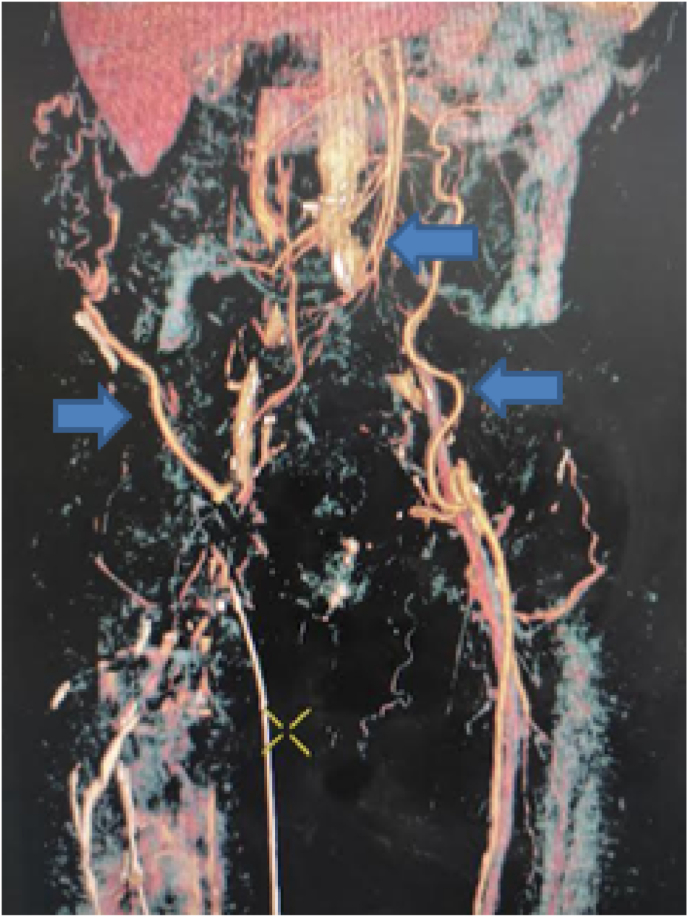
CT Angiography in coronal view volume rendering showing the presence of multiple collateral circulations (arrows).
